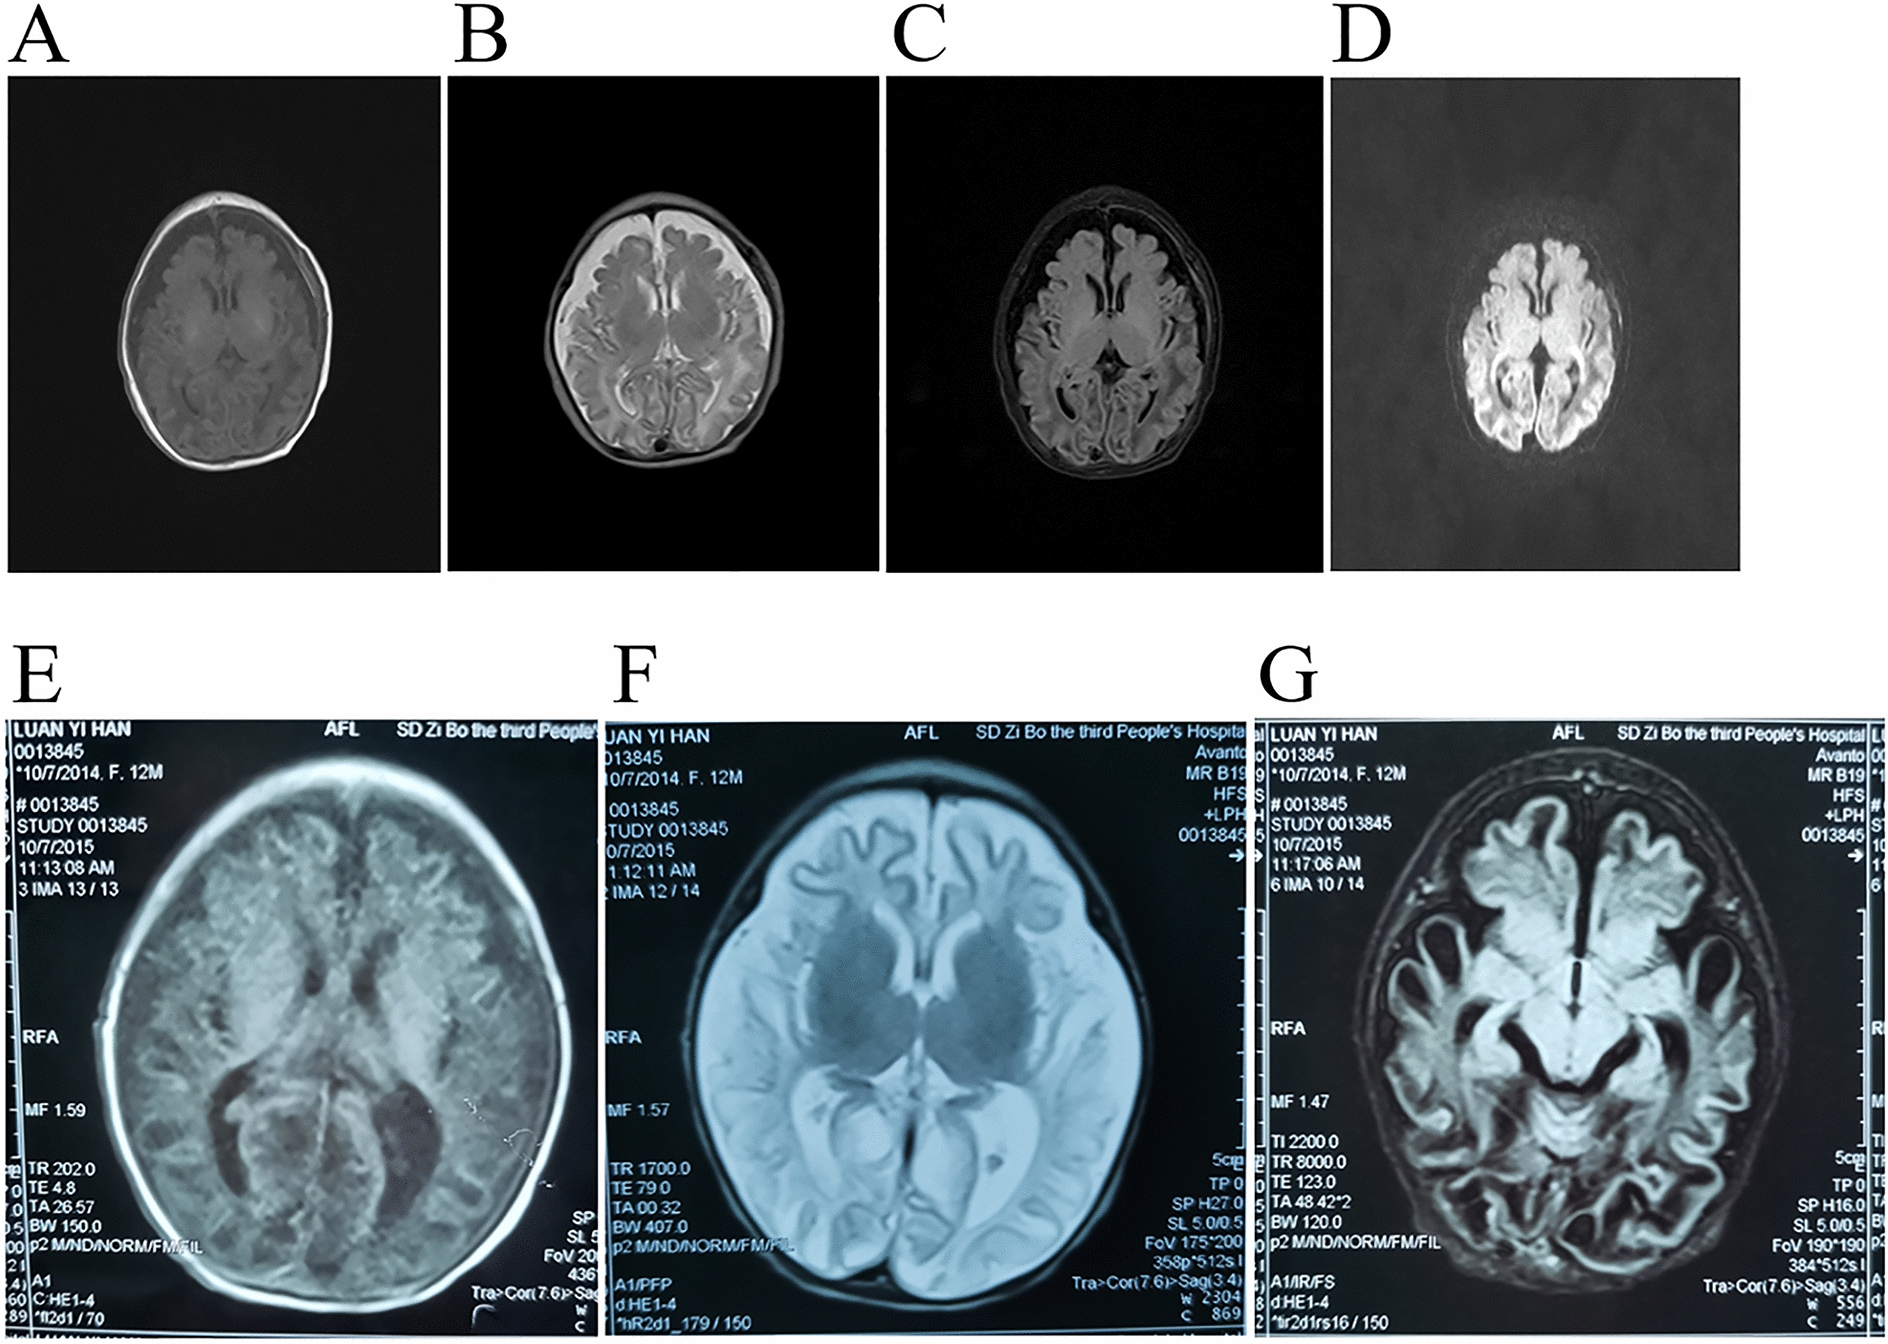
Brain MRI of the proband (A–D) and the elder sister (E–G). A and E T1-weighted image (T1WI). B and F T2-weighted image (T2WI). C and G Fluid-attenuated inversion recovery (FLAIR) image. D Diffusion-weighted image (DWI)
